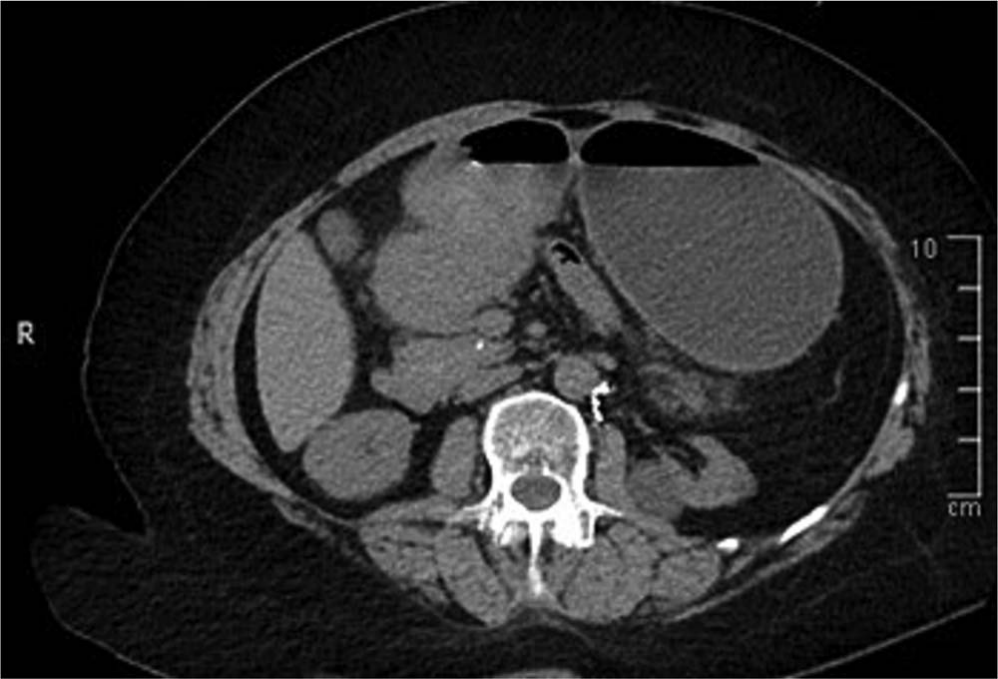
Contrast-enhanced CT of the abdomen. Axial view showing gastric outlet obstruction.
